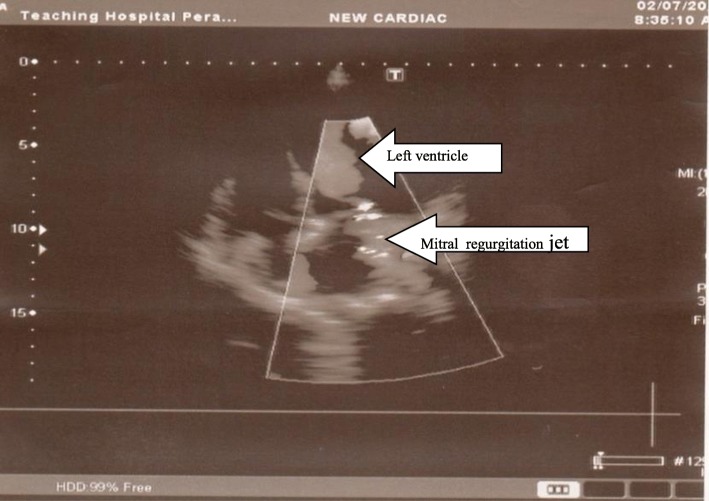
2D Echocardiogram showing mitral regurgitation
